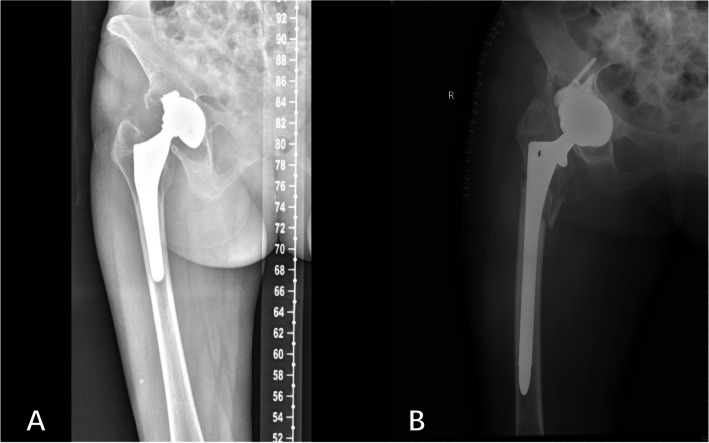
Preoperative and postoperative radiographs of cylindrical stem with intraoperative fractures
